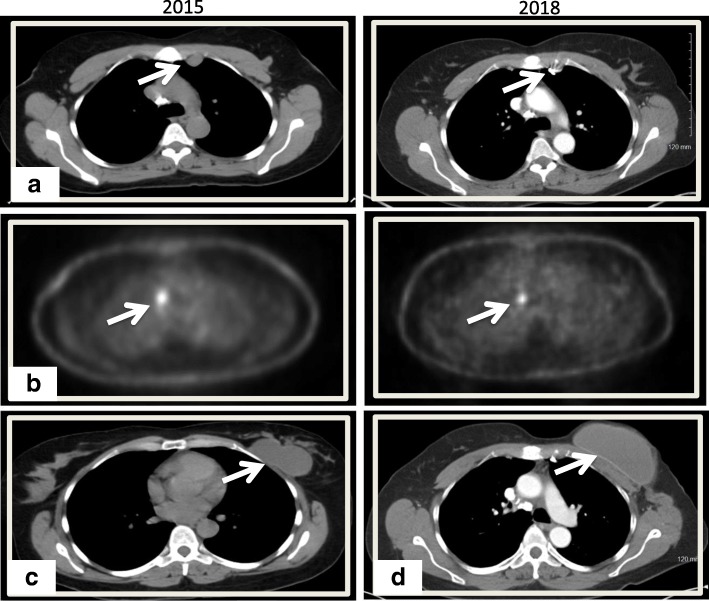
Comparison of positron emission tomographic/computed tomographic image from 2015 (prior to neoadjuvant antihormonal therapy and left breast mastectomy) versus follow-up image in 2018 (following left breast mastectomy). a Response was noted in the internal mammary lymph node following combination antihormonal therapy (*arrow*). b^18^F-fluorodeoxyglucose avid mediastinal lymphadenopathy with mild reduction in avidity following combination antihormonal therapy (*arrow*). Of note, no avidity was seen in the internal mammary lymph node, and minimal avidity surrounding the breast papillary mass was observed. c Mass in 2015 prior to antihormonal therapy and mastectomy. Note the size of this mass. d Reconstructed breast following mastectomy
